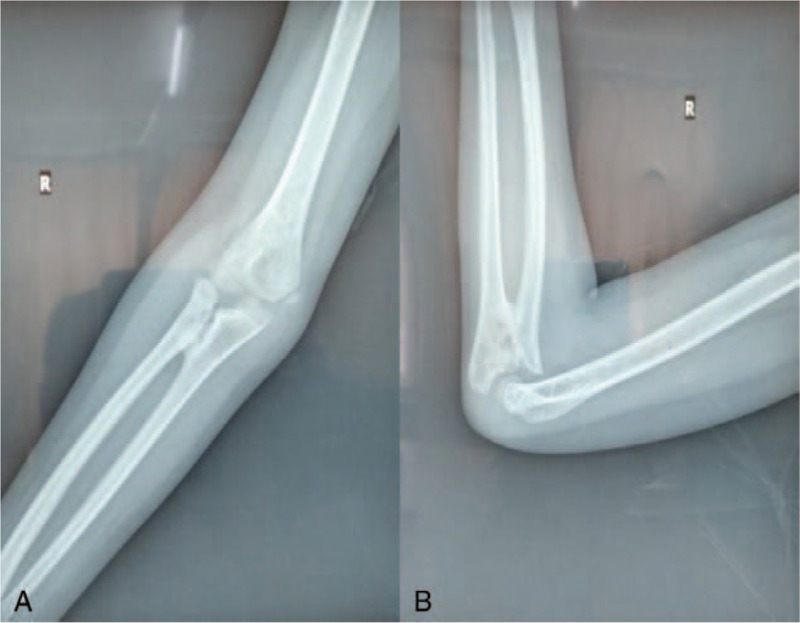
(A) X-ray of the anteroposterior elbow at the 4-mo postoperative follow-up. (B) X-ray of the lateral elbow at the 4-mo postoperative follow-up.
